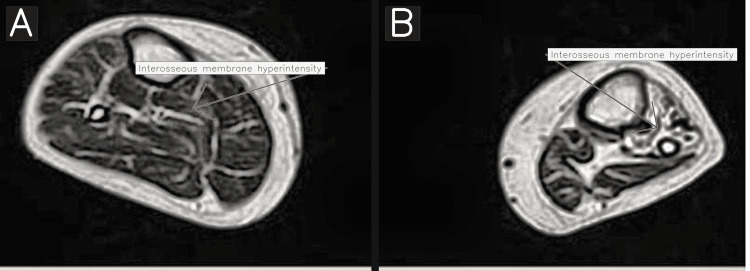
Axial MRI sequences of the left leg showing soft tissue involvement. (A) Proximal cross-section demonstrating hypotrophic anterior and lateral compartment musculature with hypointense signal areas. (B) Distal cross-section revealing hypointense regions adjacent to the fibula, consistent with mineralization within the peroneal and posterior tibial muscles.
